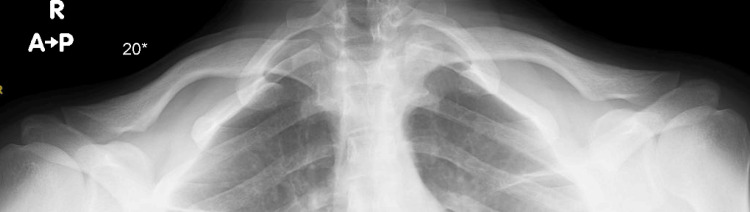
Anteroposterior-angled cephalic 20-degree X-ray of the bilateral clavicle done three months postoperatively showed reduced and symmetrical sternoclavicular joint.
